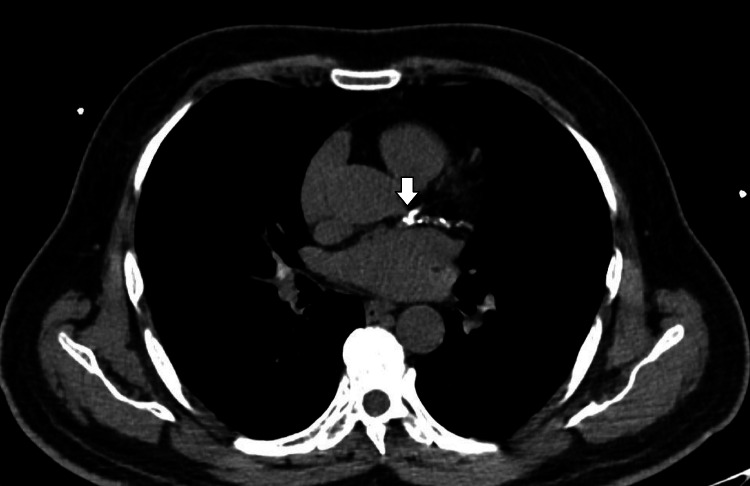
CT image of calcified LCx coronary artery The white arrow represents the lesion. LCx, left circumflex Image source: Alam et al. (2023) [10]
